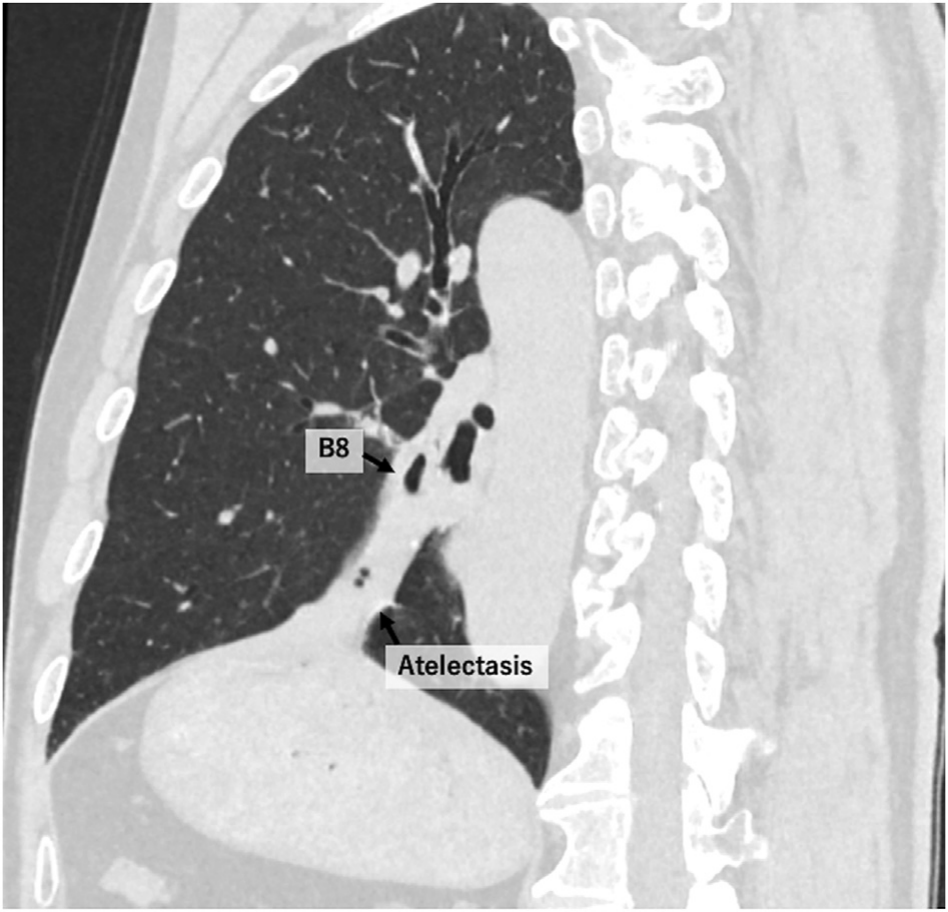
Chest computed tomography showed B8 bronchial disruption with associated peripheral atelectasis.
